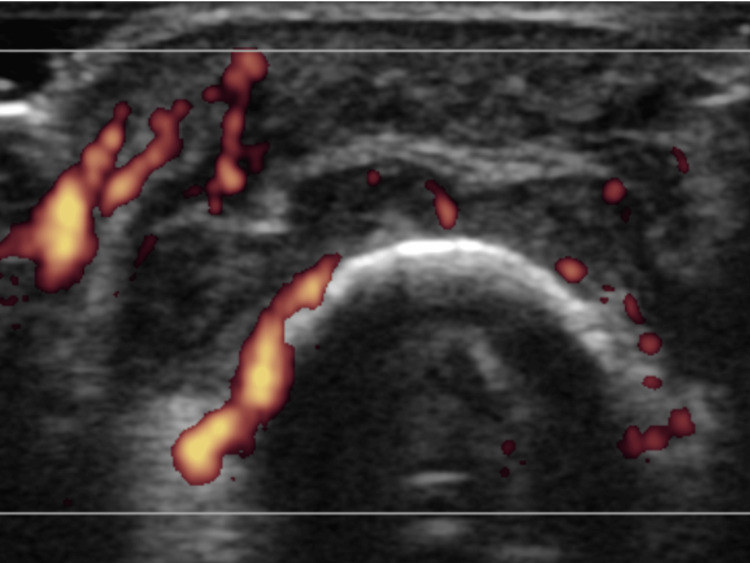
Ultrasound of the interphalangeal joint of the left middle finger showing increased vascular flow at the site of tendon attachment.
